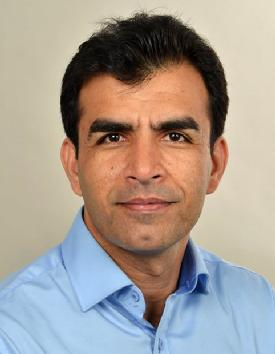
Dr Sahrai Saeed, MD, PhD, FESC is a senior consultant cardiologist and clinical researcher at Haukeland University Hospital in Bergen, Norway. His special interest includes echocardiography and multimodality imaging.
